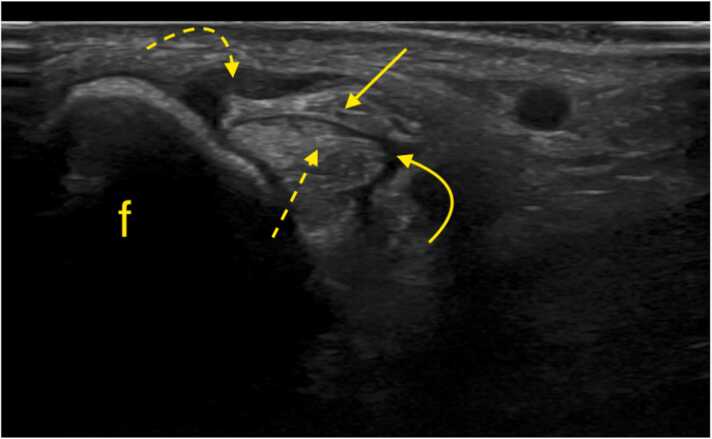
A patient with chronic pain posterior to the lateral malleolus. Ultrasound, transverse section at the level of the lateral malleolus (f), during dorsiflexion and eversion, revealed instability of the peroneal tendons. The peroneus brevis (solid arrow) is displaced above the peroneus longus (dashed arrow). The superior peroneal retinaculum appears slightly thickened (curved dashed arrow). Fluid is visible in the synovial sheath (curved solid arrow).
